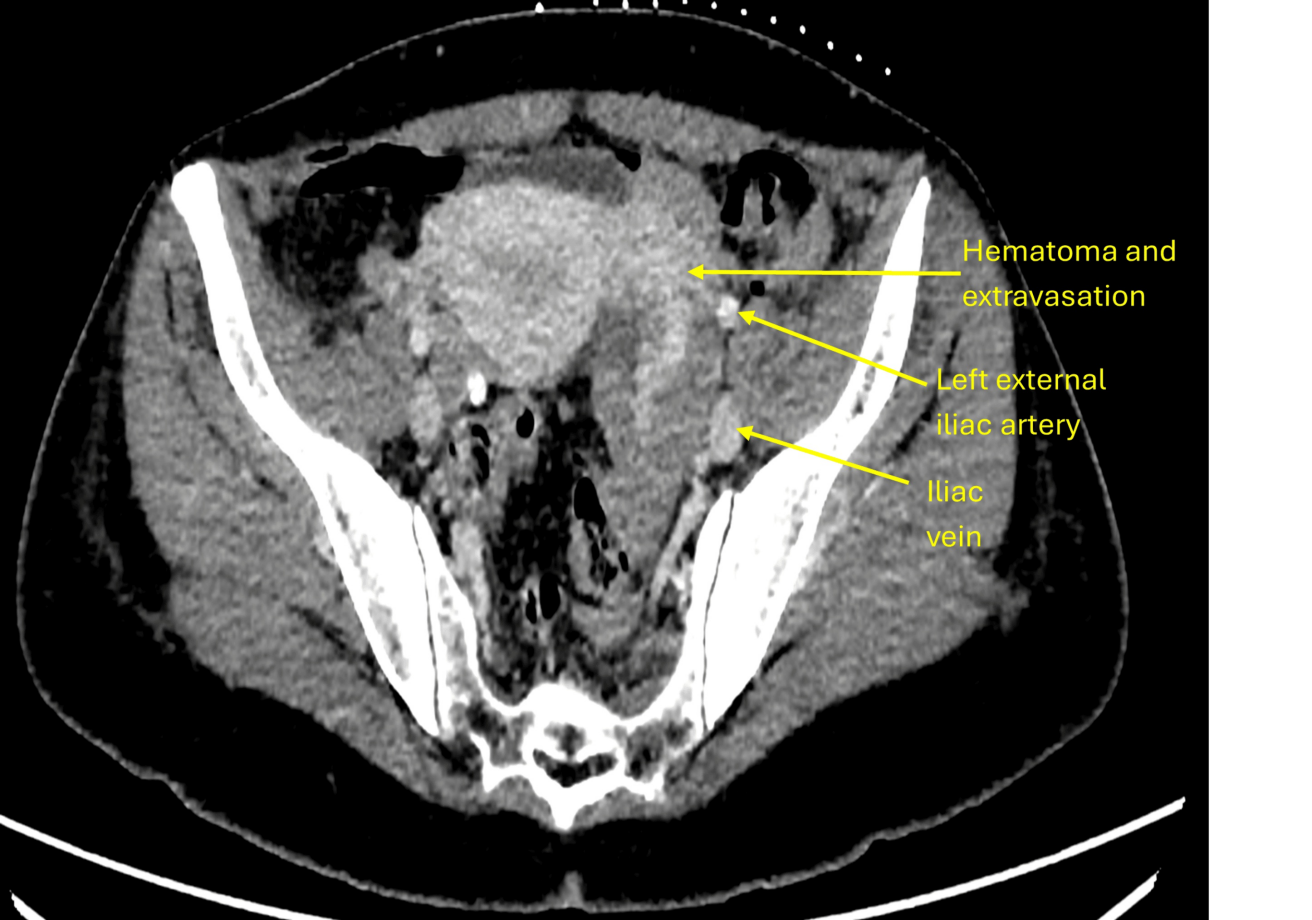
Porto-venous CT scan showing hematoma and active extravasation over the left ovarian area.
